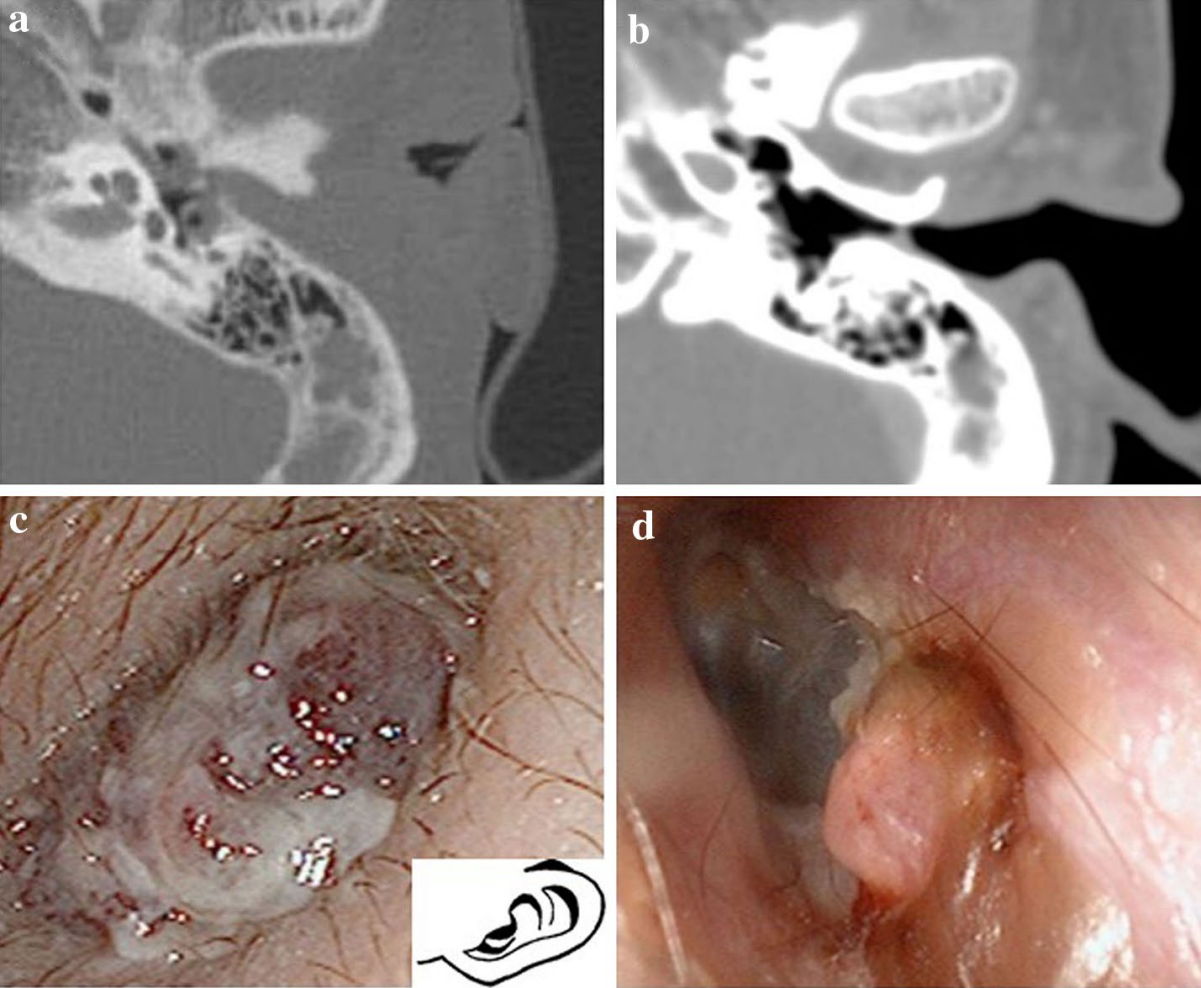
Case 3: a Soft-tissue mass filling the left auditory canal without involvement of the bony canal when he was treated for otitis media 4 months before our medical examination. b An enhanced CT scan of the temporal bone reveals an isolated enhanced soft tissue mass in the posterior-inferior wall. c Left otoscopy reveals red granulation tissue, and the tympanic membrane cannot be recognized. In later otoscopy d, the mass was markedly smaller.
